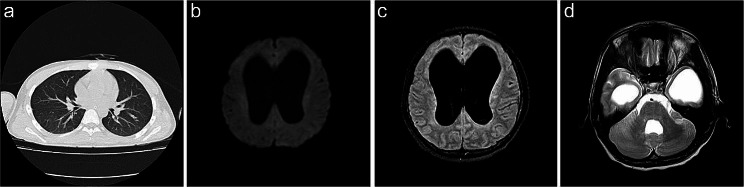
Imaging follow-up after six months of treatment reveals the disappearance of lesions in the lungs and brain. a epresents the chest CT scan; b represents the axial DWI sequence of MRI;c represents the T2Flair sequence of MRI; d represents the T2 sequence of MRI
